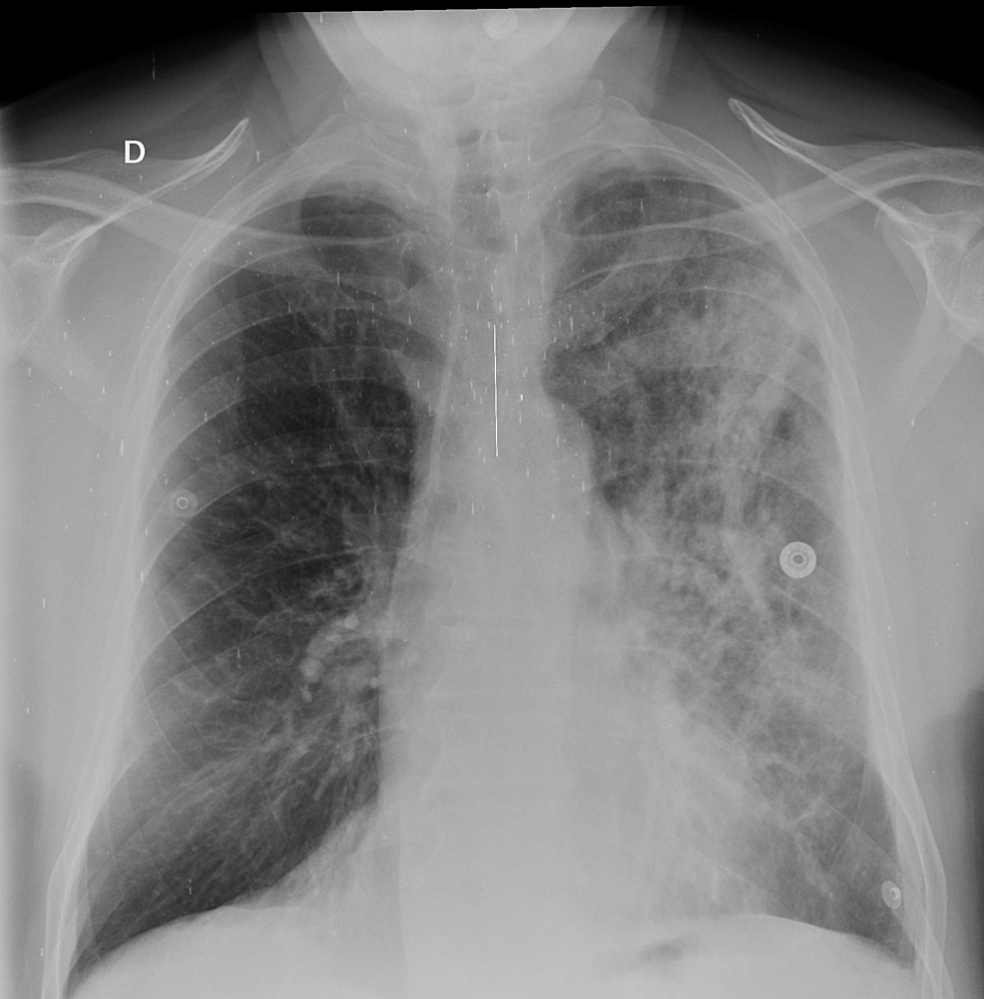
Chest X-ray showing the regression of the alveolo-interstitial syndrome in the left lung one week after the treatment
